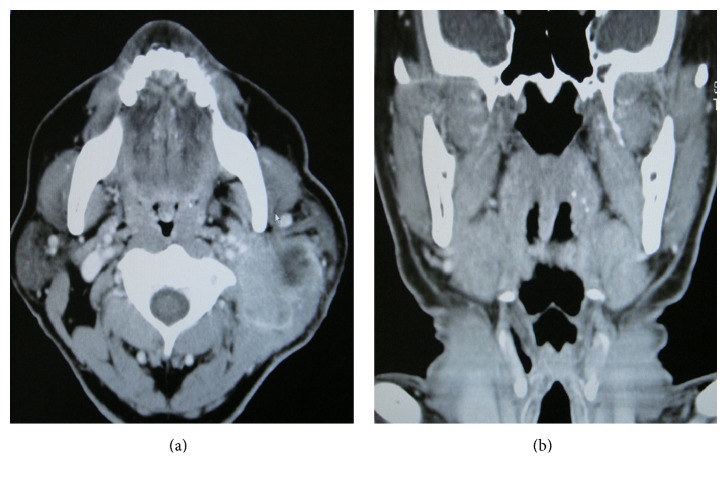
CT scan with contrast enhancement ((a) axial view; (b) coronal view). A CT scan demonstrated a cystic mass on the left side of the neck; however, tonsillar tumors were not detected.
